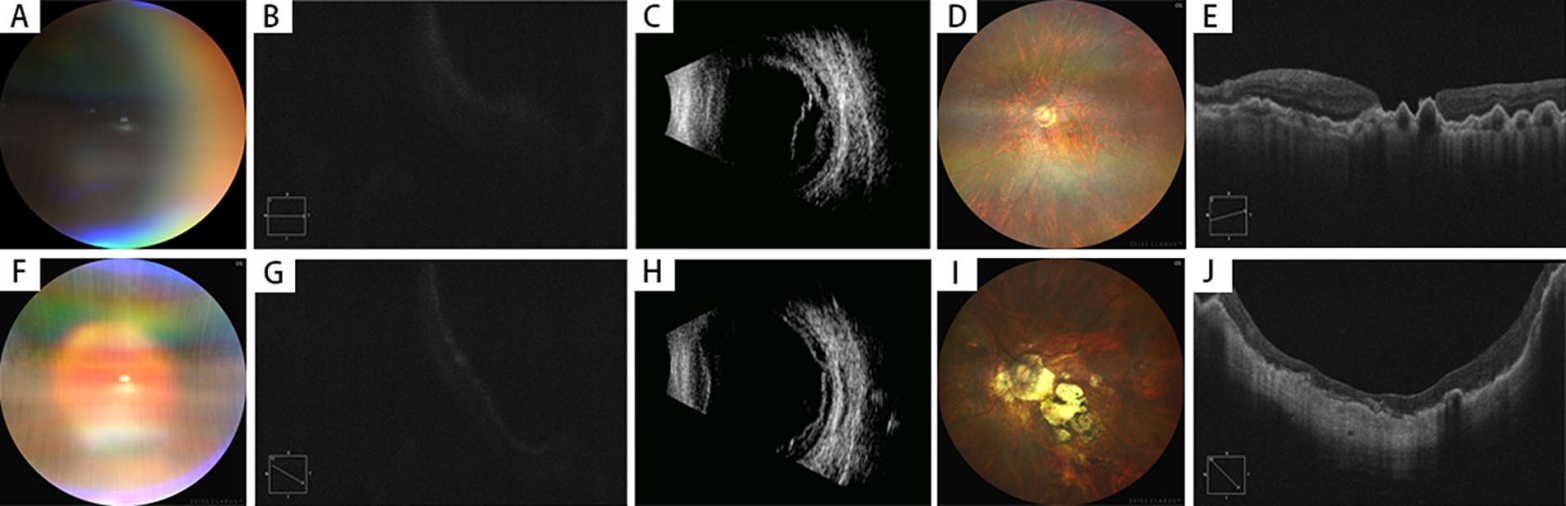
Preoperative and postoperative examinations of highly myopic eyes with macular hole retinal detachment (MHRD) with choroidal detachment (2 Cases). (A–E) Case 1 (MH Unclosed)–A 67-year-old female presented with 2 years of vision loss in her left eye, diagnosed as MHRD with choroidal detachment (A). Preoperative best-corrected visual acuity (BCVA) was 0.04 (LogMAR 1.4), and OCT could not clearly delineate the macular hole (B). B-scan ultrasonography confirmed the presence of both retinal and choroidal detachments (C). Postoperatively, the retina was reattached under silicone oil tamponade (D), but the macular hole remained open with a diameter of 1,005 μm (E). The final postoperative BCVA was 0.04 (LogMAR 1.4), and the axial length measured 29.56 mm. J: Case 2 (MH Closed)–A 64-year-old female presented with 20 days of vision loss in her left eye, also diagnosed as MHRD with choroidal detachment (F). Preoperative BCVA was counting fingers (LogMAR 1.8), and OCT imaging was obscured (G). B-scan ultrasonography confirmed retinal and choroidal detachments (H). The patient underwent an initial vitrectomy with silicone oil tamponade, but developed a recurrent retinal detachment postoperatively. A secondary surgical intervention was performed, after which the retina was successfully reattached (I), and complete macular hole closure was achieved, with a CFT of 86 μm (J). The final postoperative BCVA improved to 0.05 (LogMAR 1.3), and the axial length measured 27.6 mm.
